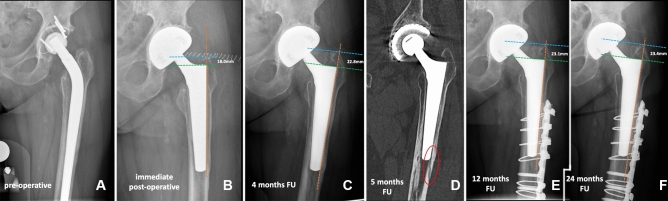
A shows a pre-operative X-ray of a long revision stem that failed due to aseptic loosening. B, C show a subsidence of 4.8 mm during the first 4 months after surgery. The patient referred to weight-bearing pain on the anterolateral side during the last three months. At the 5th month of FU, a CT scan was made due to the persistence of pain along the thigh (D), and a Vancouver type B1 fracture at the tip of the stem was detected (red ellipse). The patient was treated with ORIF with a plate, cerclages, and screws. The 12 months FU X-ray control shows complete healing of the fracture and a subsidence of 3 mm compared to the subsidence at the moment the fracture was detected (E). Almost no more subsidence was recorded 24 months after surgery (F). *FU* follow-up; *CT* computer tomography; *ORIF* open reduction internal fixation
